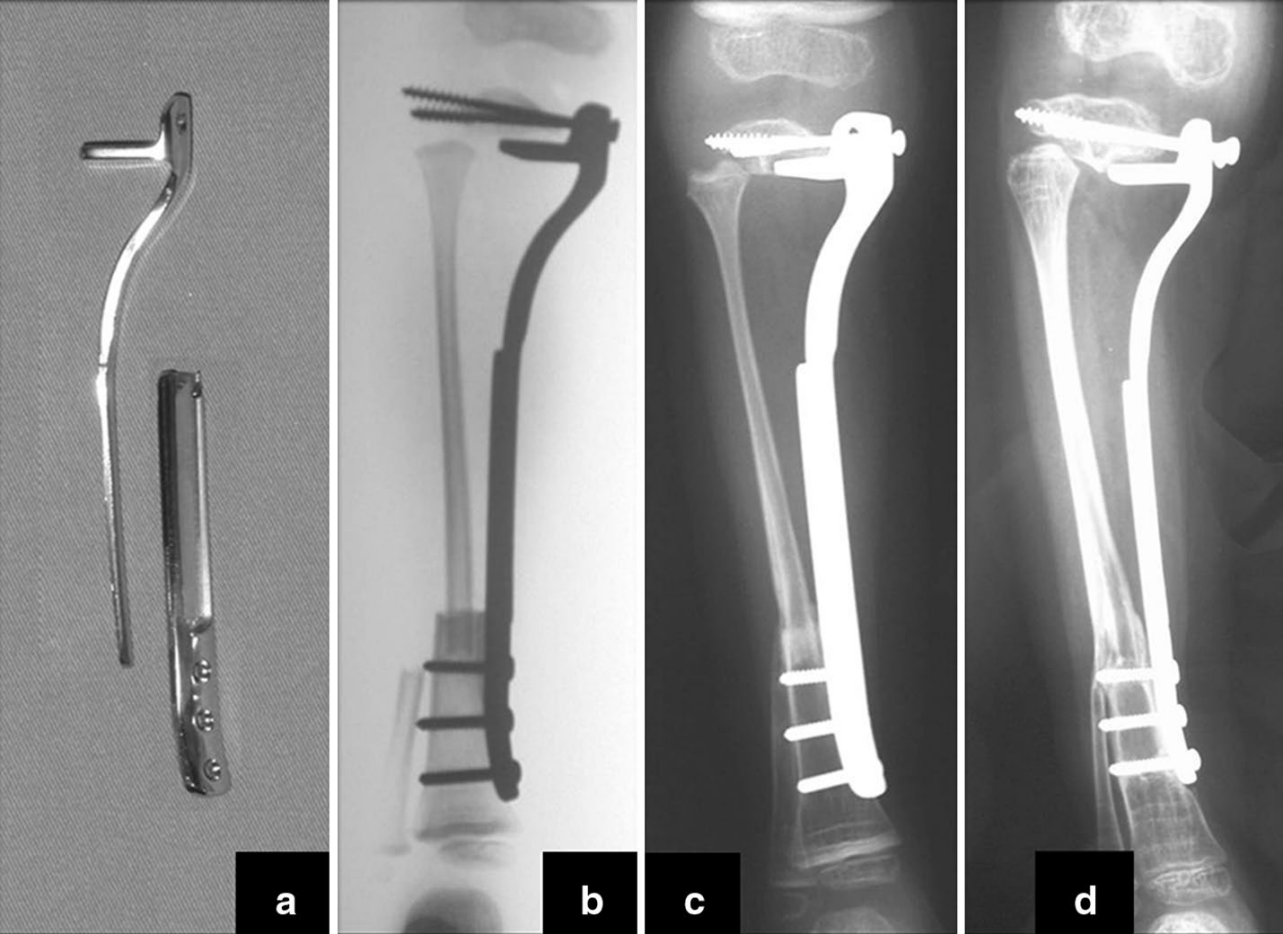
a  The proximal-plate with a horizontal support, for proximal tibia epiphisial support, b immediate postoperative radiograph, c 3 months postoperative radiograph, showing the distal tibio-fibular consolidation and d 6 months postoperative radiograph, showing hypertrophy the fibula
